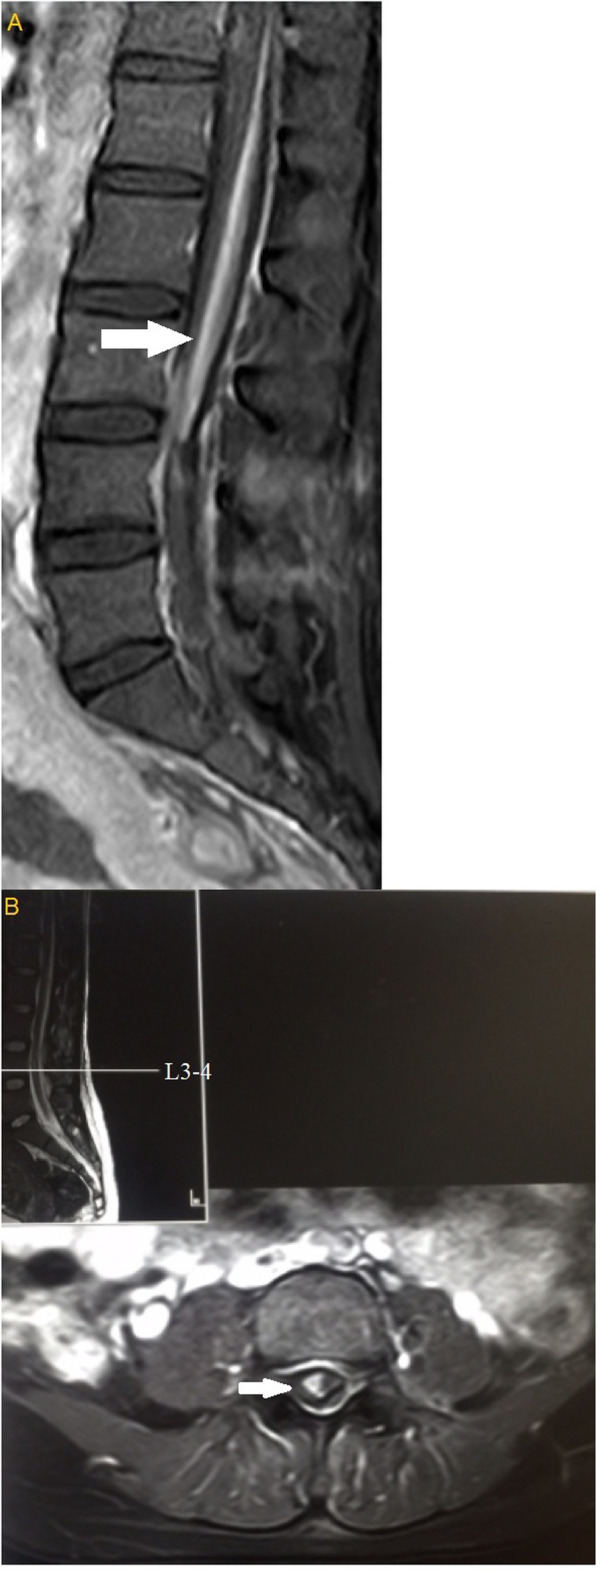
Magnetic resonance imaging of the lumbar spine after cesarean delivery (a, sagittal section; b, axial). White arrows indicate the spinal subdural hematoma
